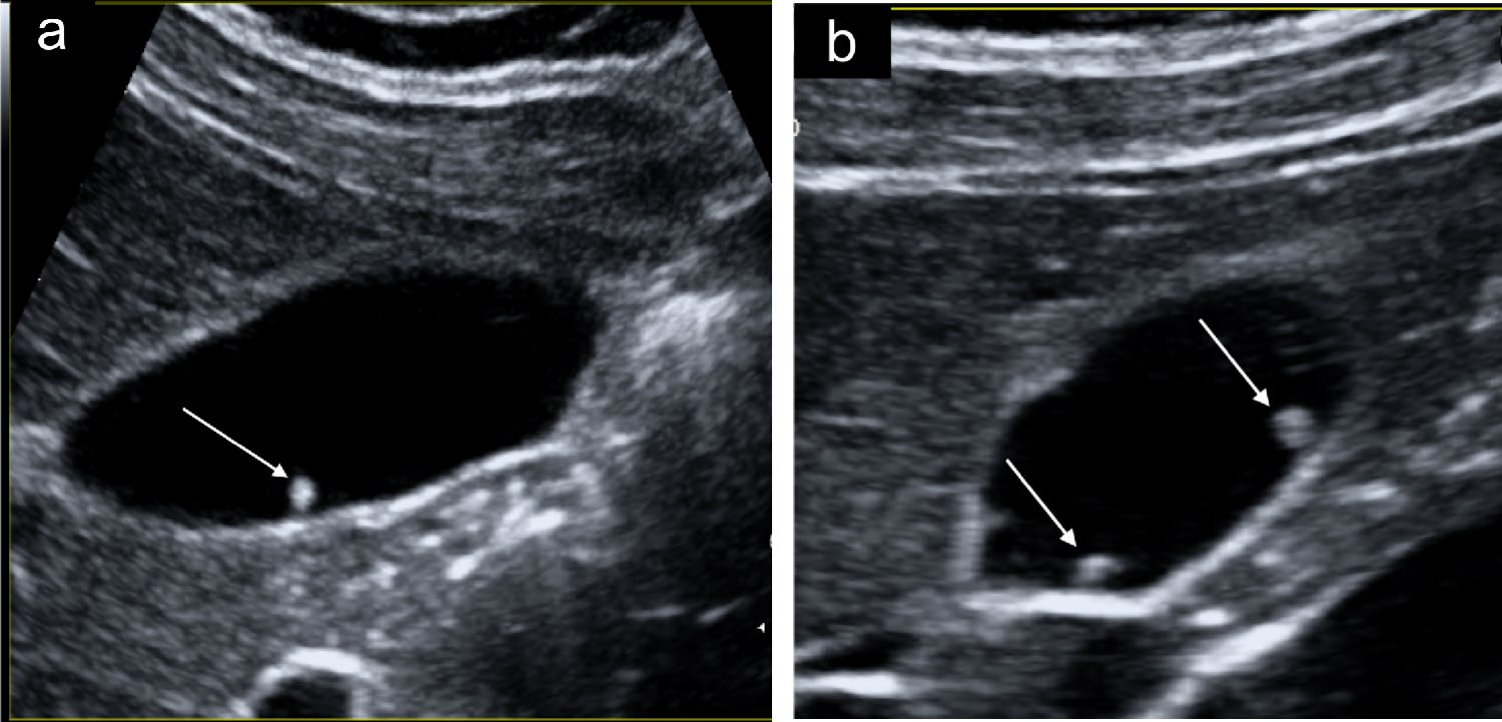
a The sonographic image shows solitary cholesterol polyps, homogeneous, pedunculated, without posterior acoustic shadowing, smaller than 1 cm, more hyperechoic than the liver parenchyma (white arrow). b The sonographic image shows two cholesterol polyps, homogeneous, pedunculated, without posterior acoustic shadowing, smaller than 1 cm, more hyperechoic than the liver parenchyma (white arrows)
